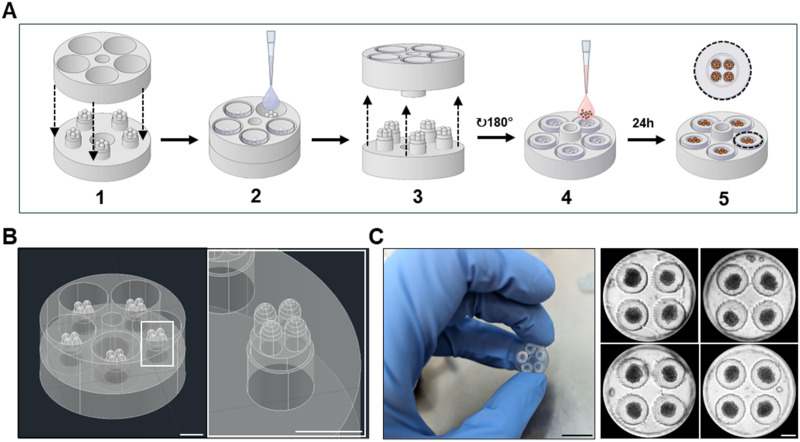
Template design provides an efficient platform for generating spheroids and assessing spheroid viability. (a) AutoCAD^®^ design of the 3D-printed template generating five individual molds, each comprising four microwells designed for spheroid formation using the ultra-low-attachment technique. (1) The top part of the template is placed onto the bottom template. (2) UltraPure™ agarose is pipetted into the template. (3) and (4) The top part is disconnected and turned over, and cells are pipetted into the molds. (5) Spheroids are formed after 24 h of seeding. (b) AutoCAD “x-ray” view showing the template used to form molds for spheroid seeding (scale bar = 2.5 mm). (c) On the left, representative images of agarose molds formed in the 3D-printed template (scale bar = 14.5 mm). On the right, Ovcar-8 spheroids formed in the generated agarose molds, 24 h after seeding 5000 cells/microwell (scale bar = 0.4 mm; n = 12).
